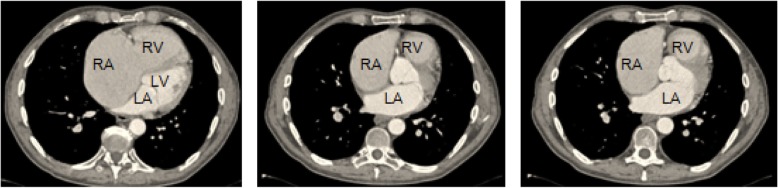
“Angio-CT - preserved continuity of the atrial septum, high degree enlargement of right atrium and ventricle”. *RA* right atrium, *RV* right ventricle, *LA* left atrium, *LV* left ventricle
